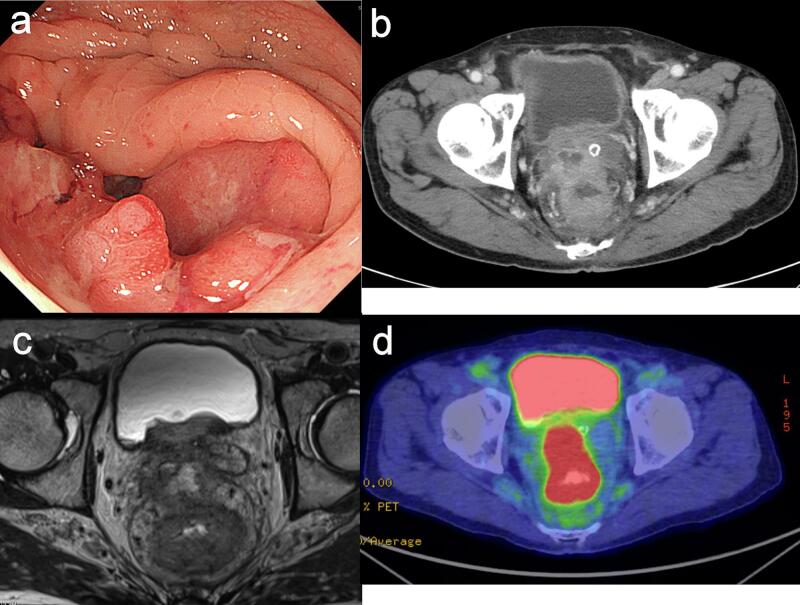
Radiological and colonoscopy findings before colostomy construction. A) Colonoscopy showing a circumferential ulcerated type tumor in the lower to upper right rectal wall. B) Abdominal contrast-enhanced computed tomography scan showing enhanced wall thickness and a lesion in the rectum, invasion of the bladder and prostate, and an abscess cavity on the ventral side of the rectum. C) Pelvic magnetic resonance imaging showing an abscess cavity on the ventral side of the rectum, bordering the bladder and prostate. D) ^18^F-fluorodeoxyglucose (FDG)-positron emission tomography/computed tomography showing FDG uptake in the rectal tumor and abscess.
